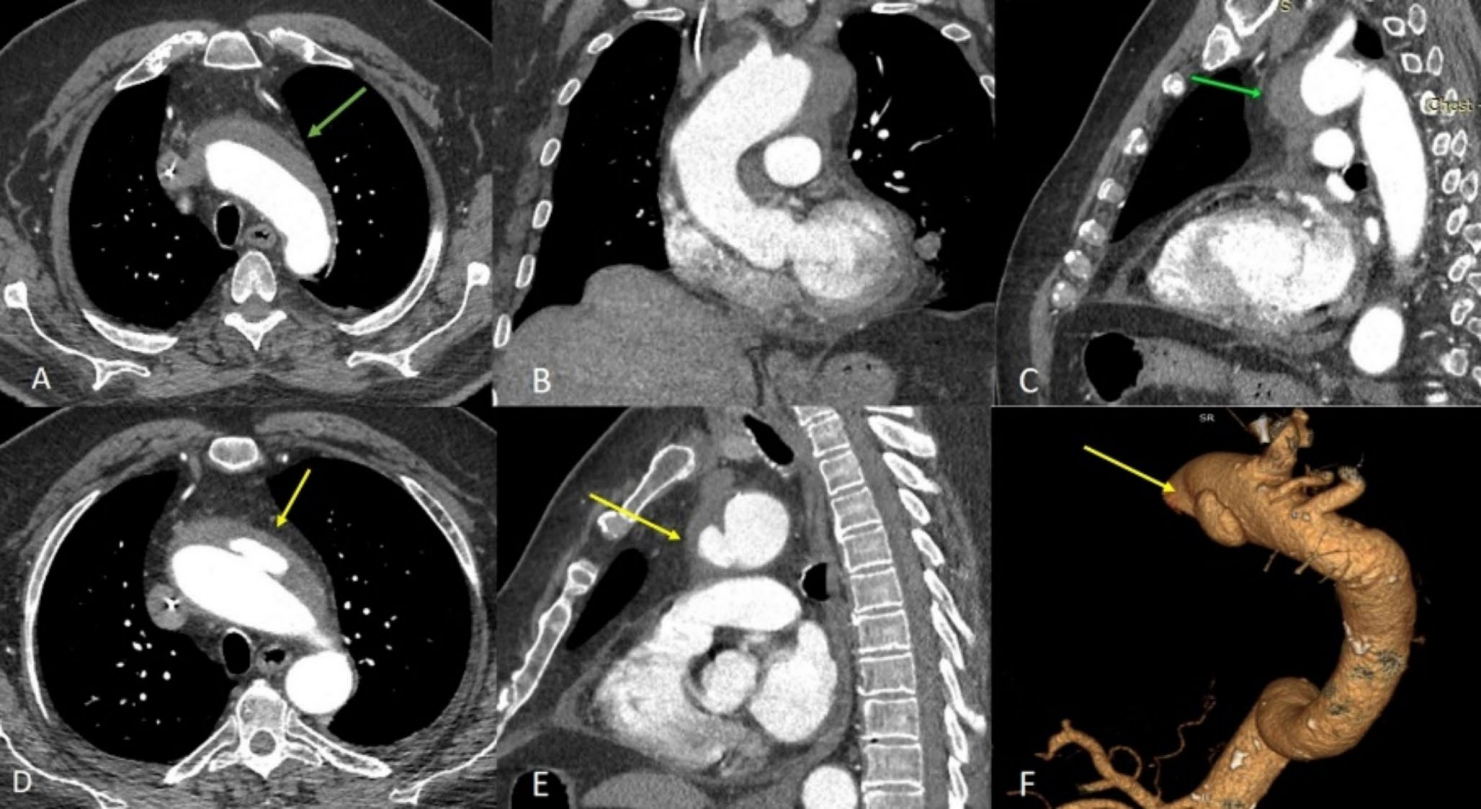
Intramural hematoma with an ULP (ulcer-like projection). Axial (A) coronal (B) and sagittal (C) computed tomography angiography (CTA) images of an intramural hematoma of the thoracic aorta (green arrows). Axial (D) and sagittal (E) images of the thoracic aorta at the site of the coexisting ULP. Post processing with volume rendered image of the ULP (D)
